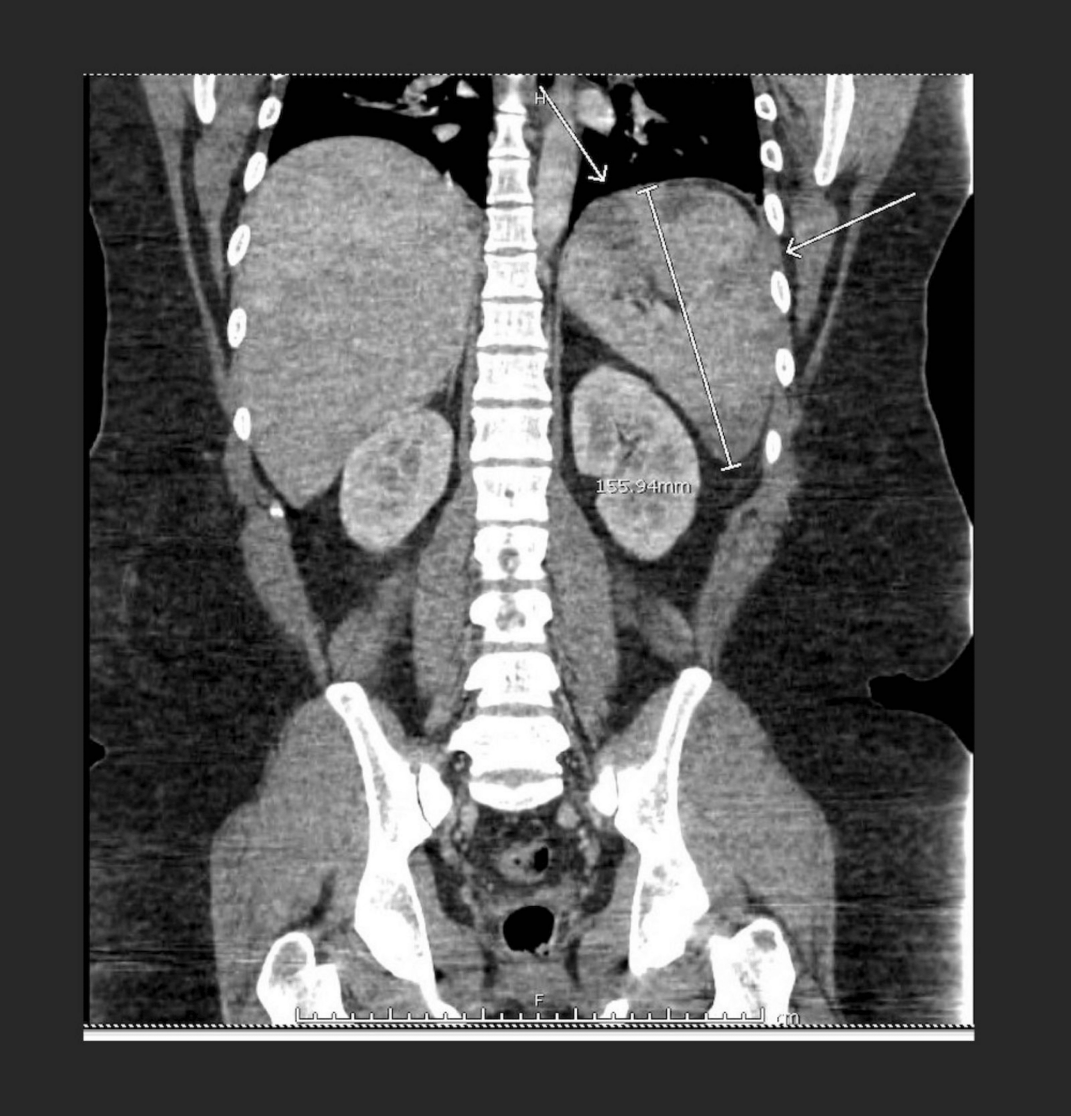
Coronal CT image demonstrates marked splenomegaly (white arrow) with multiple wedge-shaped hypodense areas consistent with splenic infarcts CT: computed tomography
